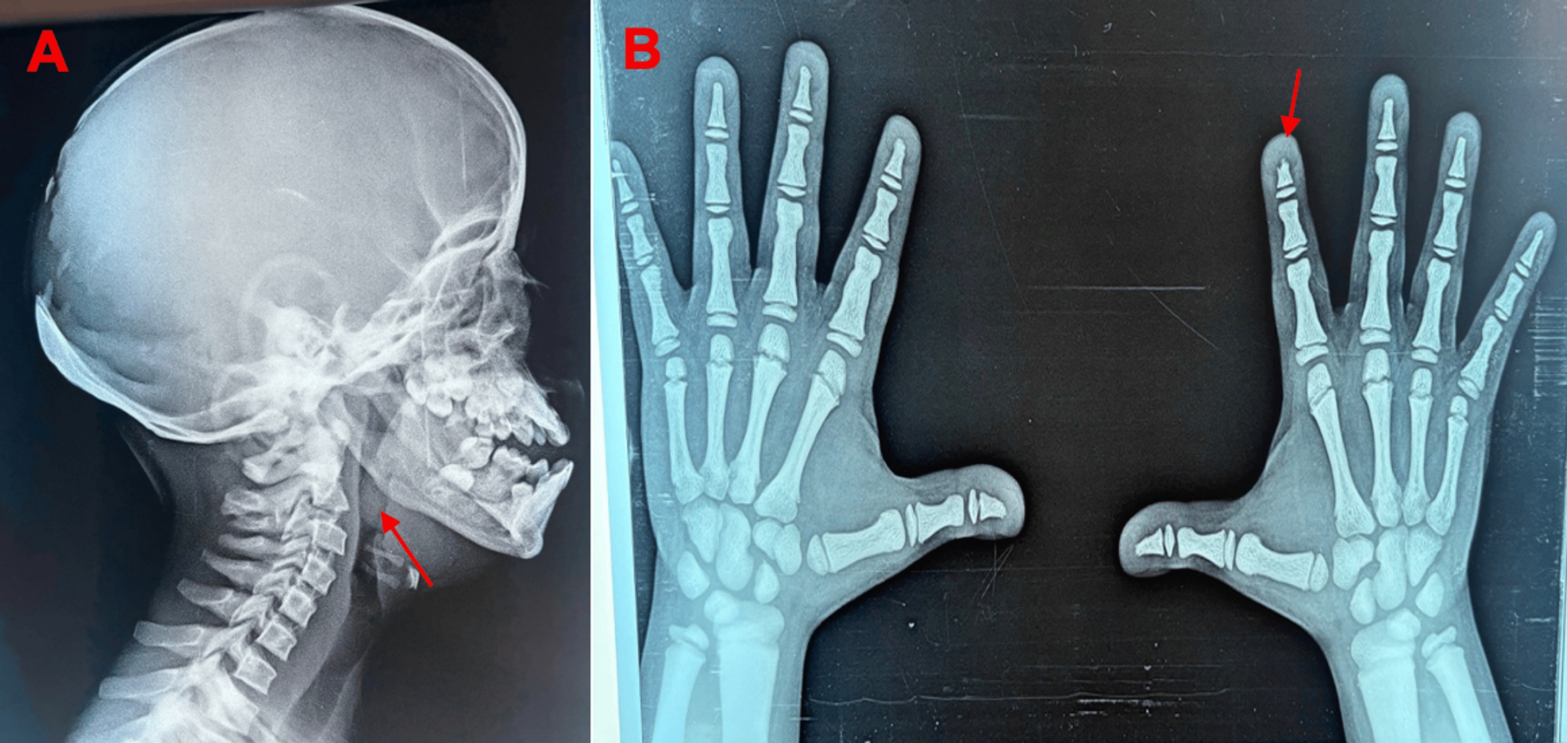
(A) Red arrow showing the obtuse angle of the mandible and (B) bilateral hand X-ray showing a short distal phalanx
